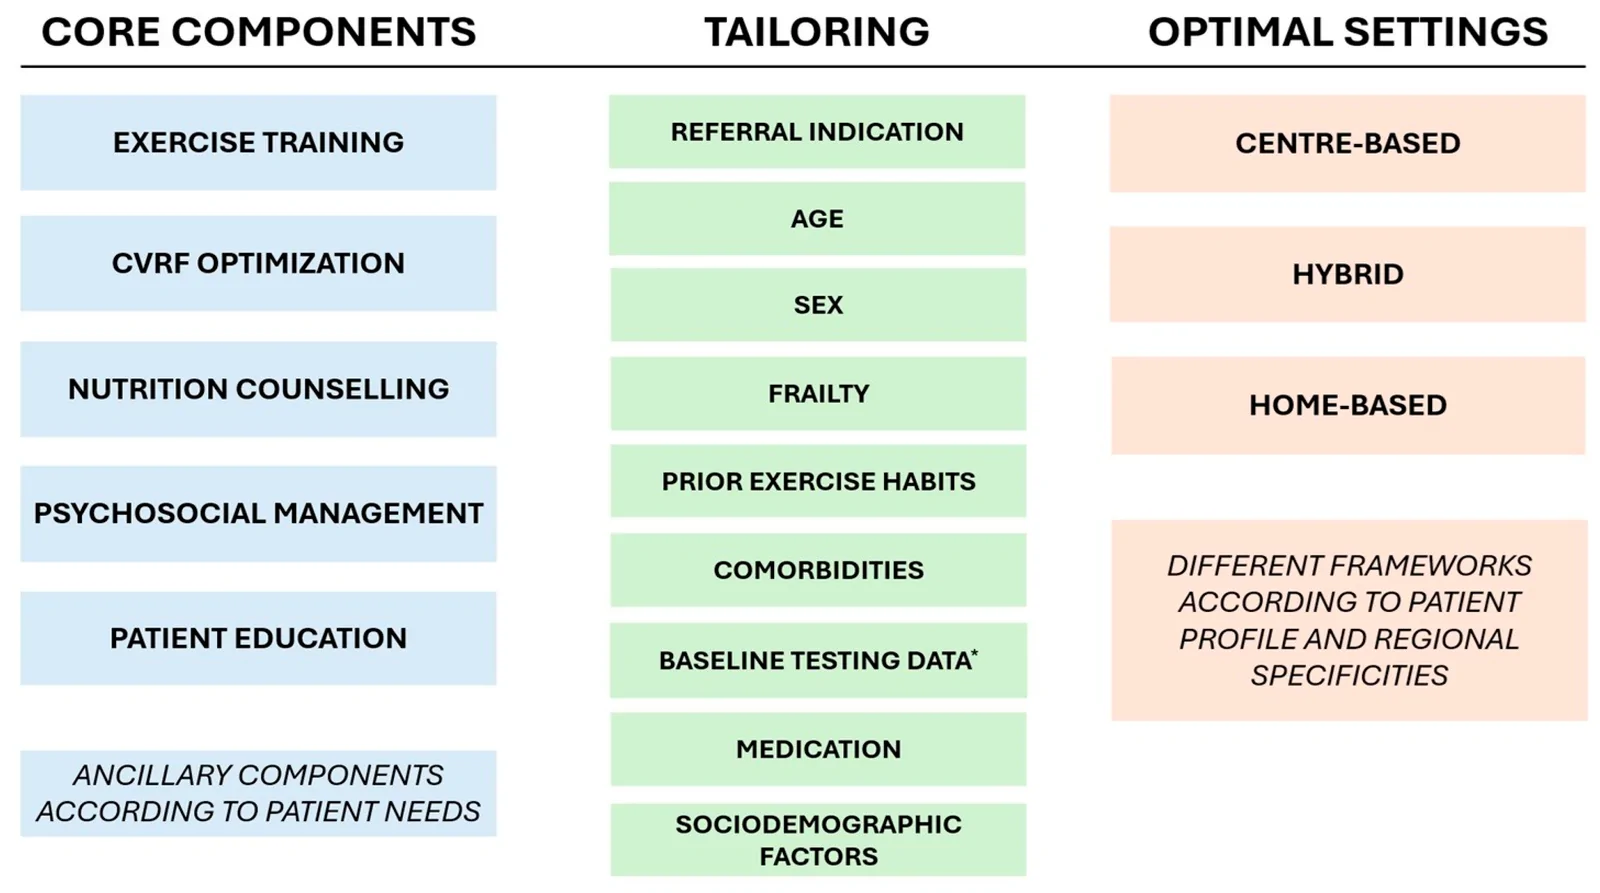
Cardiac rehabilitation—from general core concepts to personalized management. Contemporary cardiac rehabilitation (CR) programmes comprise a multidisciplinary structured intervention, encompassing several components. An individualized assessment and tailoring of programme components according to patient characteristics is a central part of CR. To tackle some of the barriers to CR implementation such as transportation difficulties, lack of CR centre coverage, or time constraints, frameworks such as hybrid or home-based programmes have also gained increased recognition (see main text for further details). CVRF, cardiovascular risk factors; *, including exercise stress testing, echocardiography and biochemical parameters.
